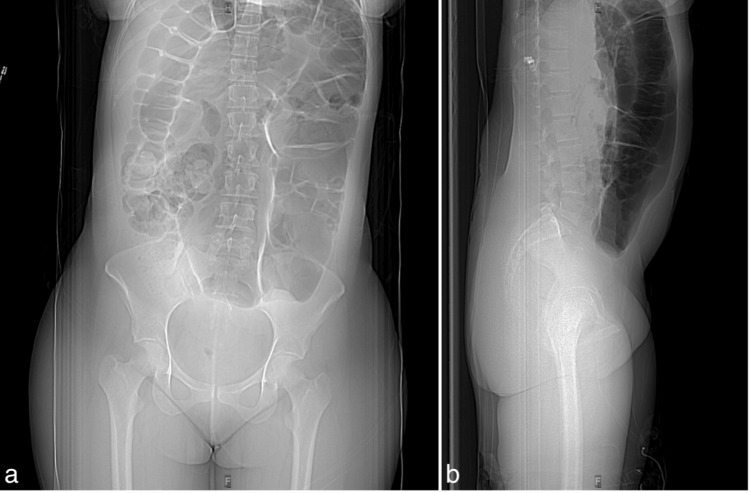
CT abdomen, low dose without IV contrast (Revolution CT, GE Healthcare®) Coronal (a) and sagittal (b) scout images showing an air-filled distended sigmoid colon. CT: computed tomography, IV: intravenous
